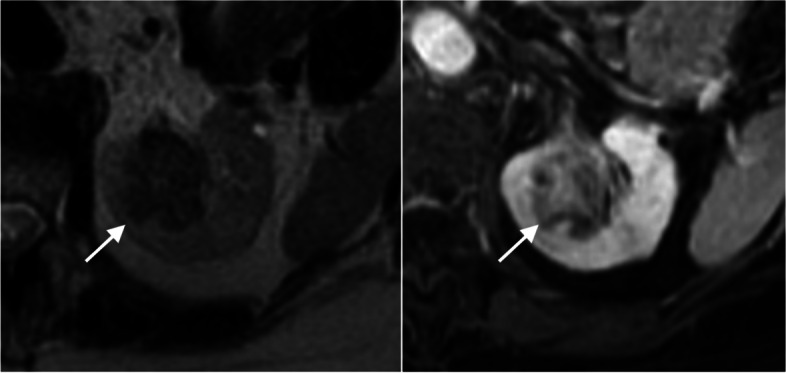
75-year-old woman with sarcomatoid RCC showing large volume of T2LIA. T2-weighted axial image (A) shows 3.7-cm left kidney endophytic mass (arrow) manifesting entirely with T2LIA (volume of 11.4 mL; 100%) that enhances on post-contrast T1-weighted image (B). Bone scan showed metastasis to left femur at baseline (not shown). Cytoreductive radical nephrectomy was done after which pathological analysis showed a 5.5-cm collecting duct type carcinoma with extensive sarcomatoid features and renal sinus fat invasion. Patient died 83 days after surgery before commencing further treatment. RCC = renal cell carcinoma; T2LIA = T2 low signal intensity area
